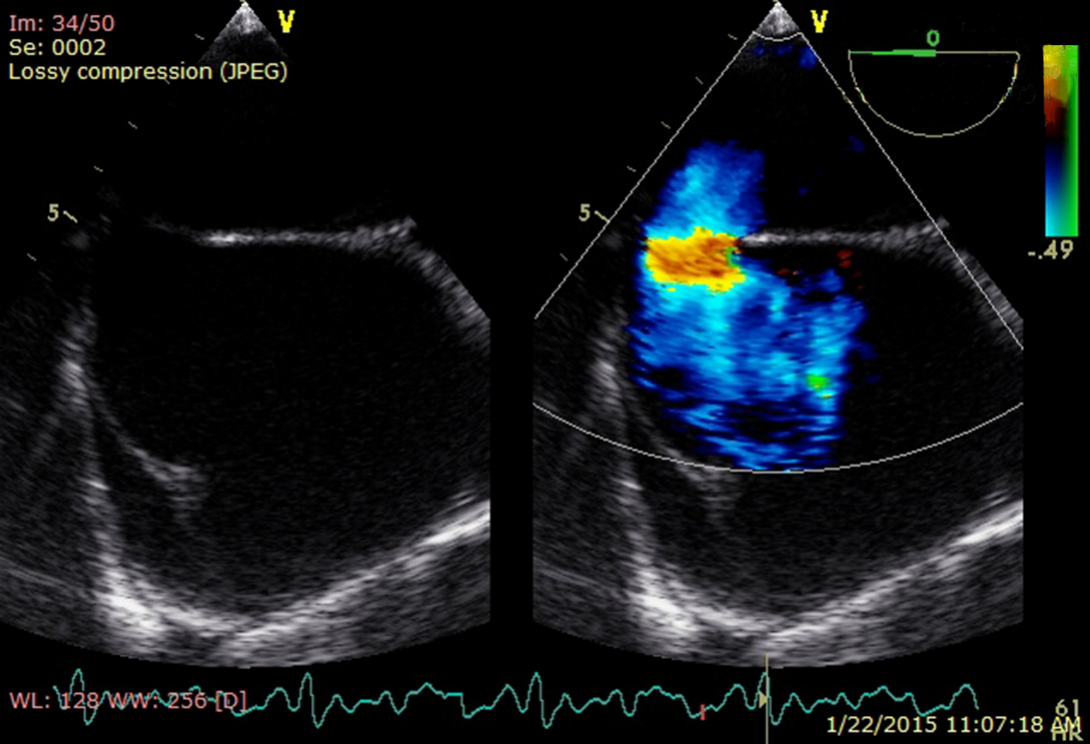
Transesophageal echocardiography showing Atrial Septal Defect sinus venosus type.
